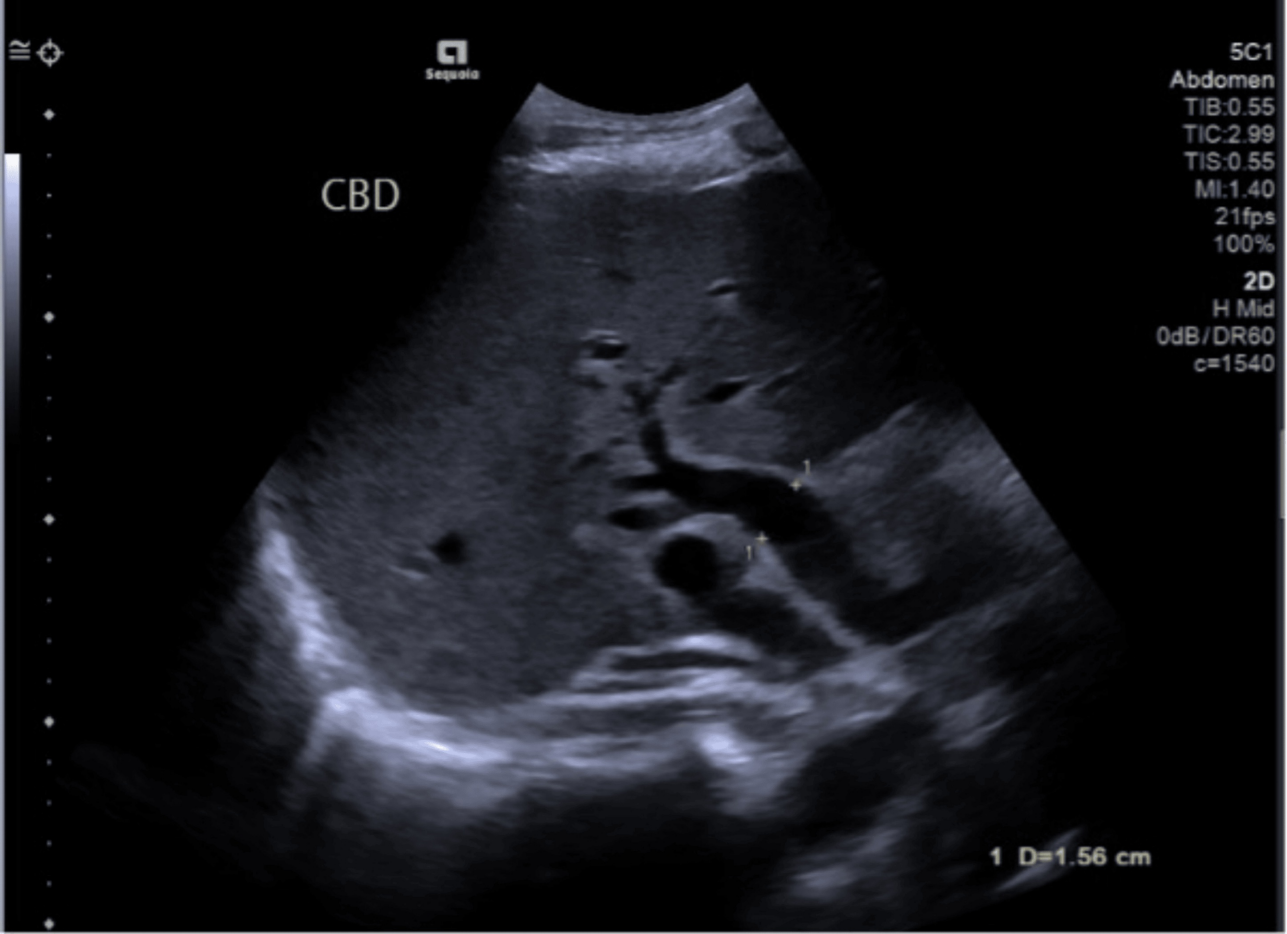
An ultrasound of the abdomen showing measured CBD dilatation with no calculi CBD: common bile duct
